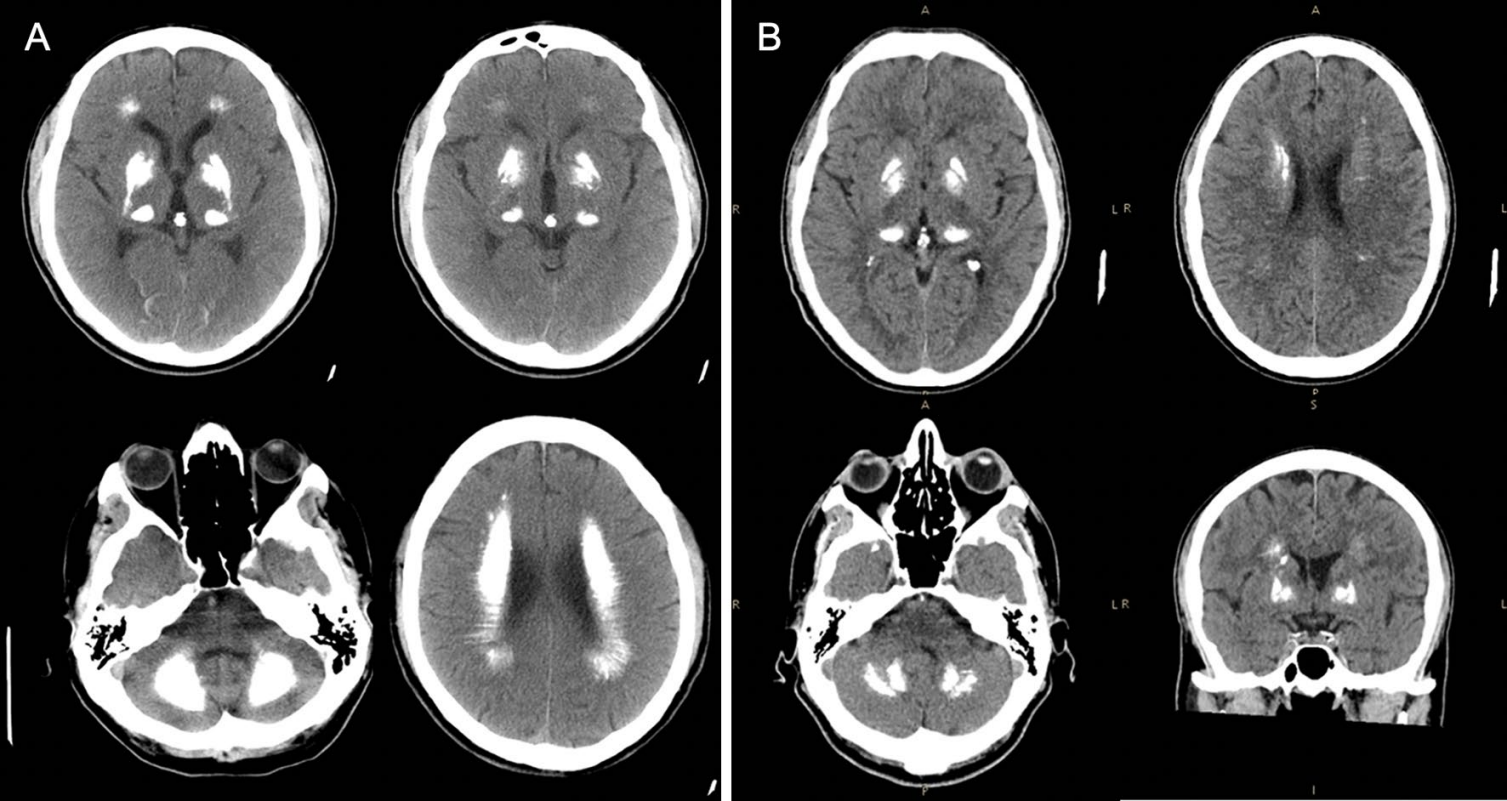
Brain CT scan of: A a patient carrying a pathogenic *SLC20A2* mutation with extensive bilateral calcifications of the putamen, globus pallidus, thalami, cerebellar dentate nuclei, subcortical white matter and occipital cortex; B a patient carrying a pathogenic mutation of *PDGFRB* with calcifications of globus pallidum interna, thalami, cerebellar dentate nuclei and with mild involvement of the subcortical white matter
